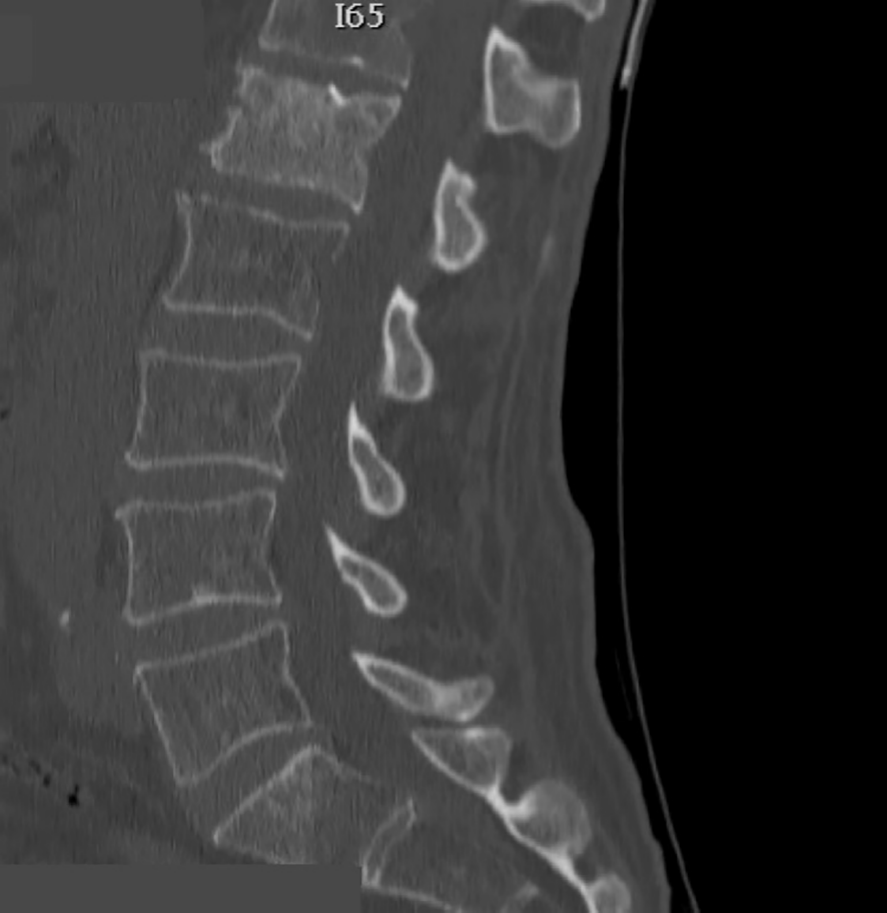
Sagittal thoraco-lumbar CT scan showing L1 metastatic lesion with altered bone density and osteolytic areas.
